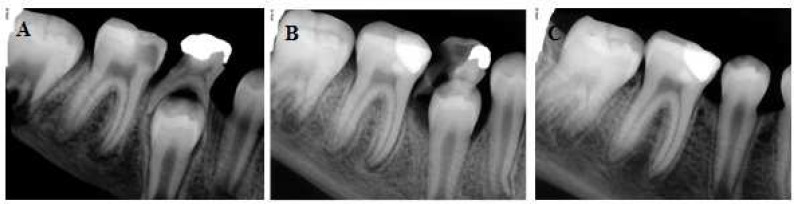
A)* Preoperative image of the mandibular right molar with involved vital pulp and immature apices; *B*) post-operative radiographic image of the tooth after performing VPT using Biodentine; *C*) Follow-up radiography and apical maturation
